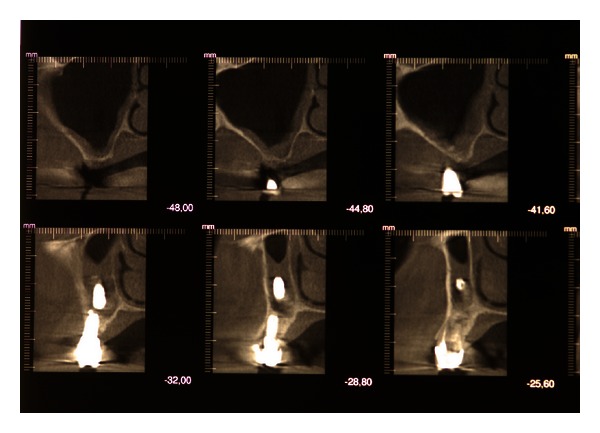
CBCT scans showing a dental implant displaced into the maxillary sinus.
